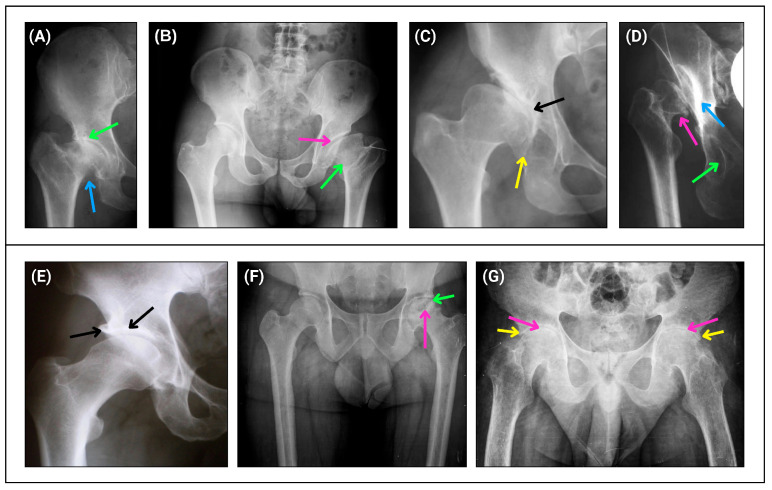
Radiographic representations of the Crowe (A–D) and Tonnis (E–G) hip classifications of dysplasia cases. (A) The green arrow indicates a reduced joint space with a reduced femoral head in the acetabulum, and the blue arrow indicates a short femoral neck (Crowe 1 and no subluxation). (B) The pink arrow indicates a femoral head with loss of acetabular coverage and the green arrow indicates a short femoral neck (Crowe 2 and low dislocation). (C) The black arrow indicates a previous acetabular roof with advanced degenerative changes due to abnormal contact between the femoral head and the acetabulum, and the yellow arrow indicates a previous acetabular roof (Crowe 3 and low dislocation). (D) The blue arrow indicates a new acetabulum due to abnormal contact between the parts, the green arrow indicates the previous/native acetabulum, and the pink arrow indicates a dislocated and deformed femoral head (Crowe 4 and high dislocation). (E) The black arrow on the left indicates an osteophyte and the black arrow on the right indicates a sclerosis of the acetabular roof (Tonnis 1). (F) The pink arrow indicates a cyst in the femoral head, and the green arrow shows a moderate reduction in the joint space (Tonnis 2). (G) The pink arrow indicates advanced joint space reduction and the yellow arrow indicates an osteophyte in the acetabular roof (Tonnis 3).
